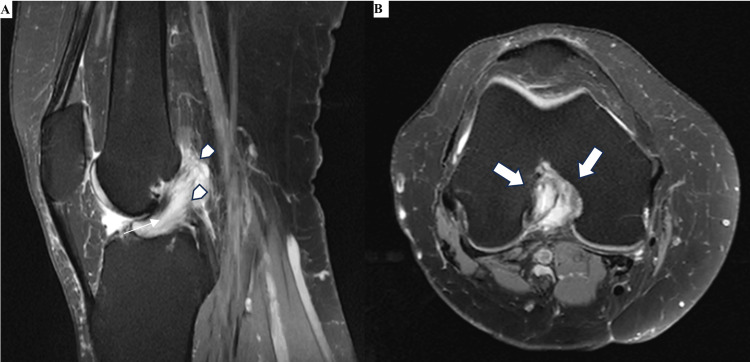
MR images of the right knee showing direct signs of mucoid degeneration of the anterior cruciate ligament MR images of the right knee (A) Sagittal PD-FS image: celery stalk sign with preserved fibers (arrowheads) and interstitial high-signal material (arrow), parallel to the Blumensaat line. (B) Axial PD-FS image: crescent-shaped appearance of the hypertrophied ACL (arrows) filling the intercondylar notch. PD-FS: proton density fat-saturated; ACL: anterior cruciate ligament
